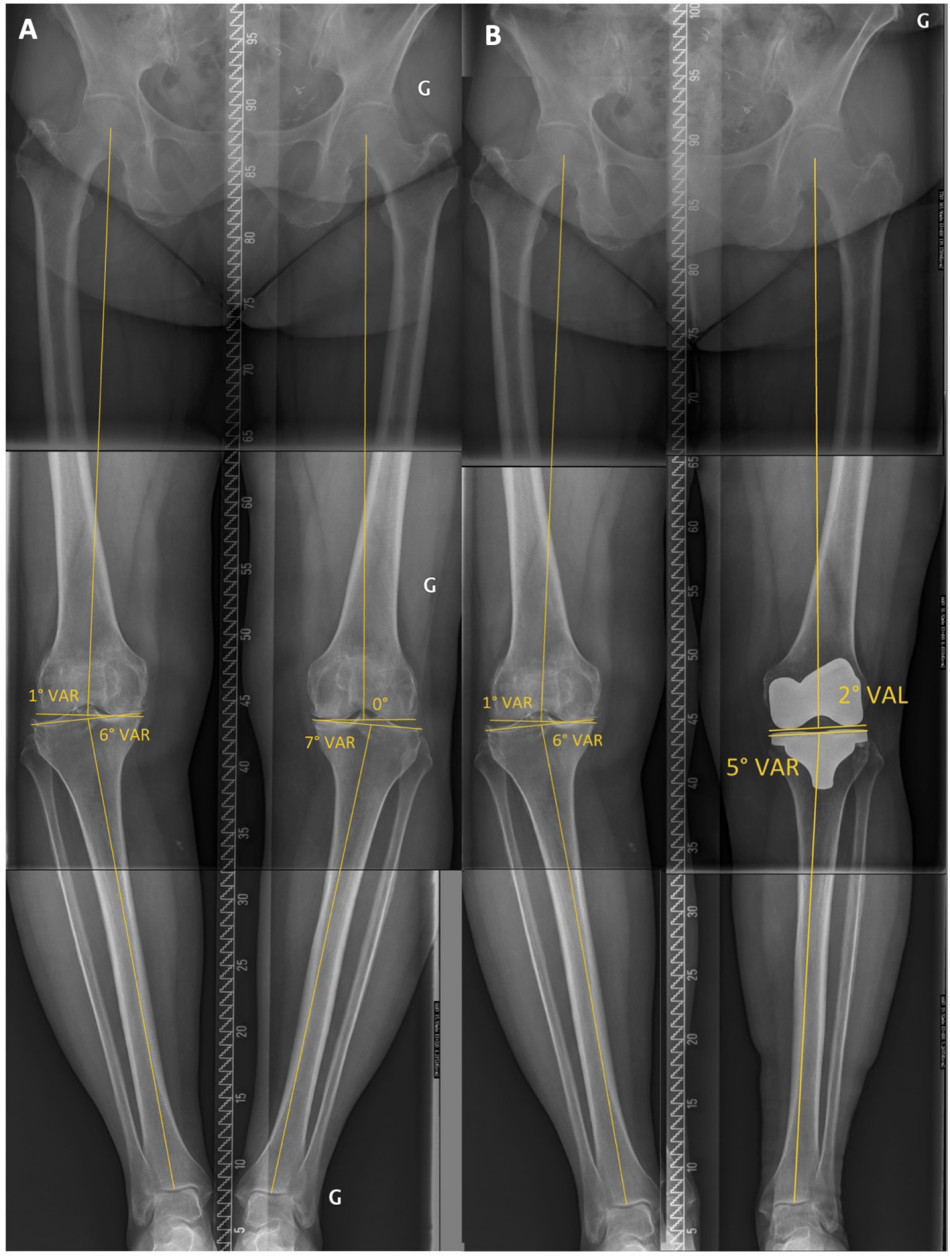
Case example 1, (A) preoperative standing long radiograph of a patient with a left lower limb mDFA of 0° and a mPTA of 7° varus, resulting in an aHKA of 7° varus. (B) on the postoperative radiograph, following the rKA algorithm, the implants mDFA is 2° valgus and mPTA is 5° varus.
